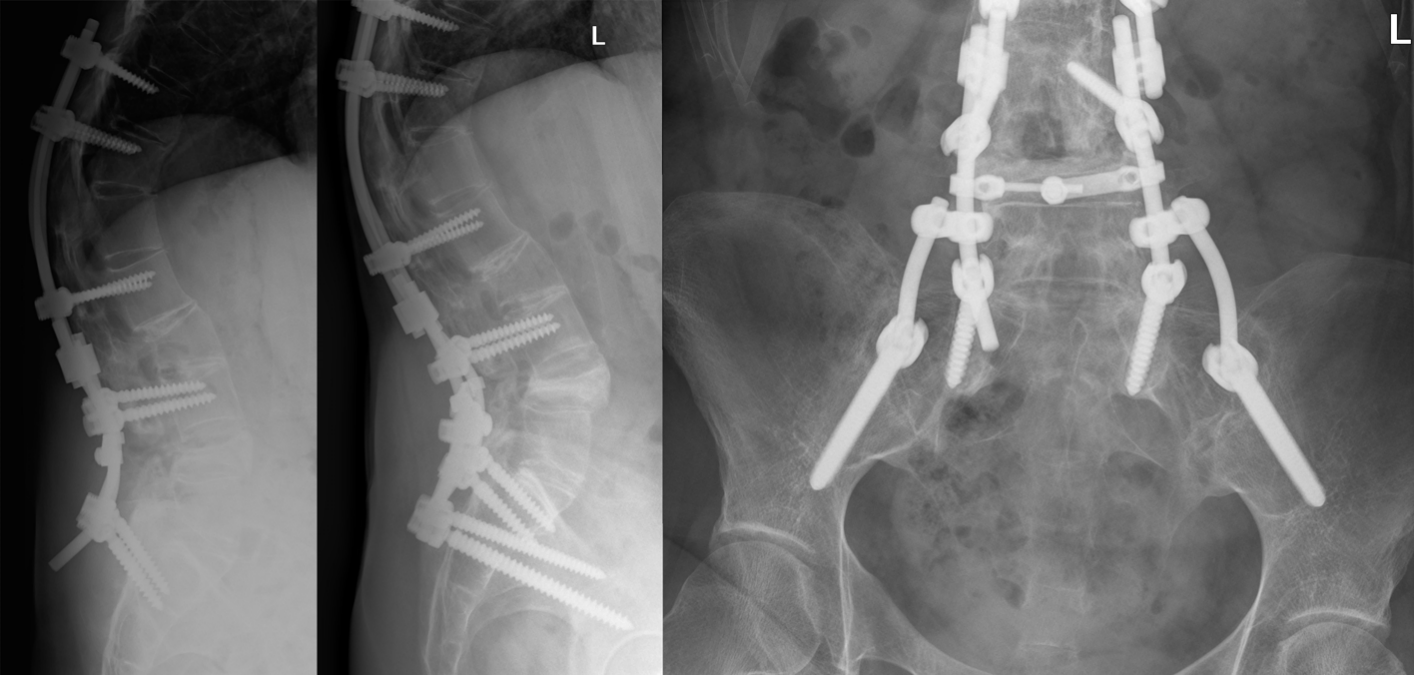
Case 3. A 44-year old woman with a progressive thoracolumbar kyphotic deformity caused by ankylosing spondylitis was treated by a 40 degree closing wedge lumbar osteotomy at level L4. Unfortunately, the pedicle screw fixation in S1 broke out, resulting in loss of deformity correction at L4. Revision surgery was performed to restore the deformity correction with bilateral iliac screws in a FB construct. Figure 4a: Pre-operative lateral radiograph. Note the loss of correction and S1 screw failure. Figure 4b: Postoperative lateral radiograph. Figure 4c: Postoperative anteroposterior radiopgraph.
